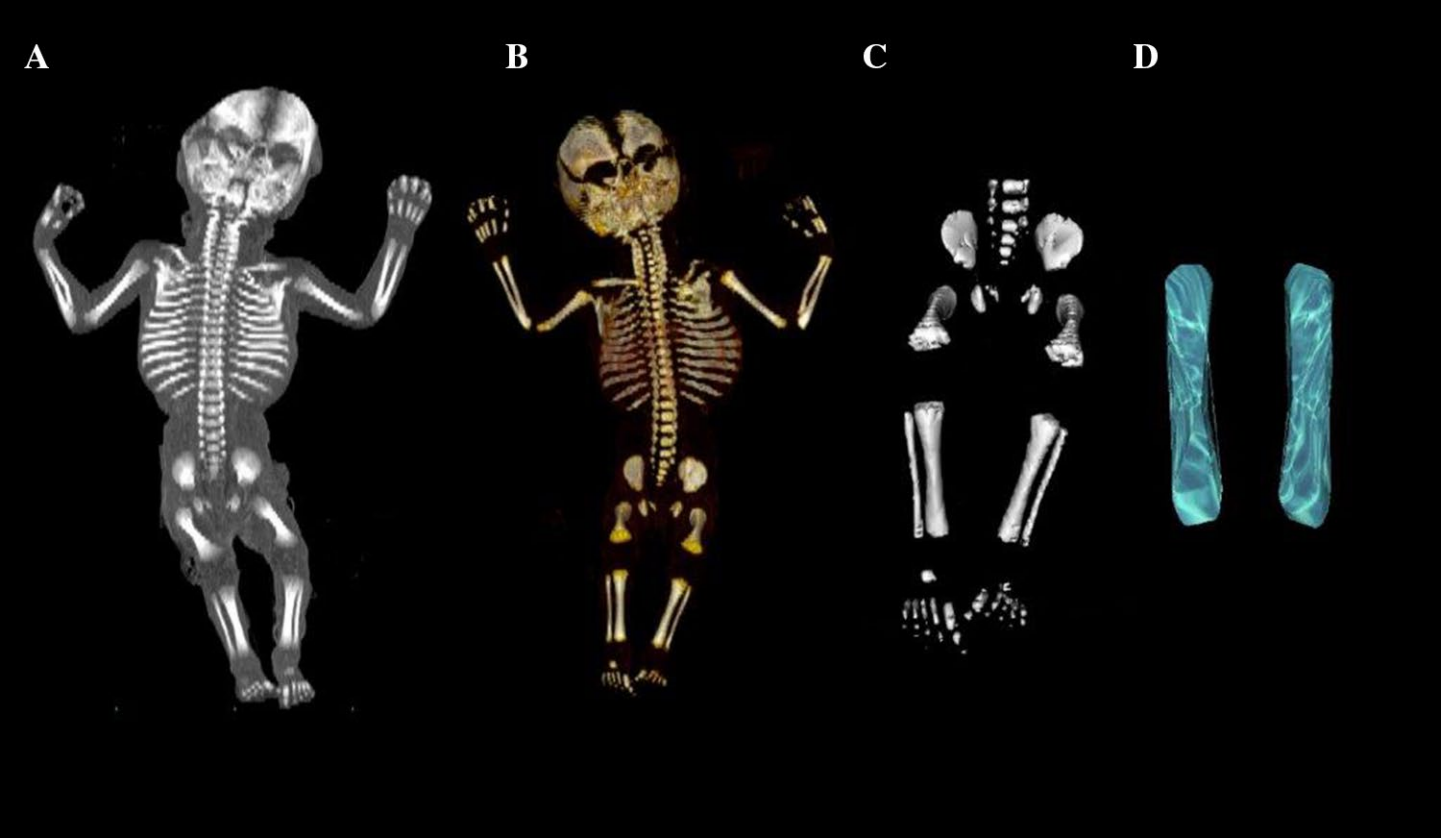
CT of a male fetus aged 19 weeks (in the frontal projection) recorded in DICOM formats (a), with the frontal reconstruction of fetal bones (b), with the frontal projection of the fetal pelvic girdle, and right and left lower limbs (c), with 3D reconstructions of ossification in the right and left tibial shafts (d) of the fetus, assessed by Osirix 3.9
